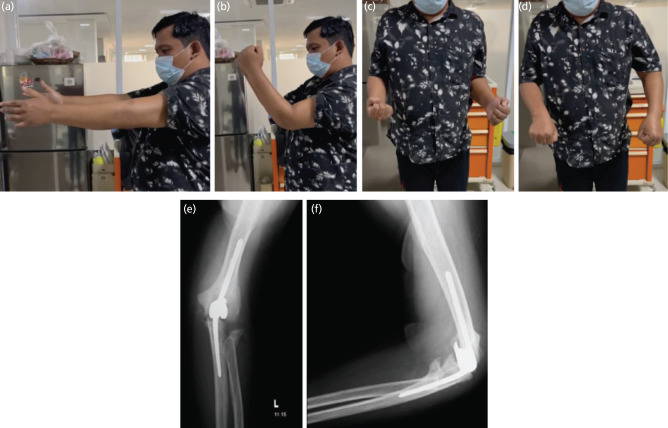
(a, b, c, d) Clinical condition one year post-operatively and radiograph evaluation showing implant in good condition with no sign of loosening, (e) in AP and (f) lateral view.
